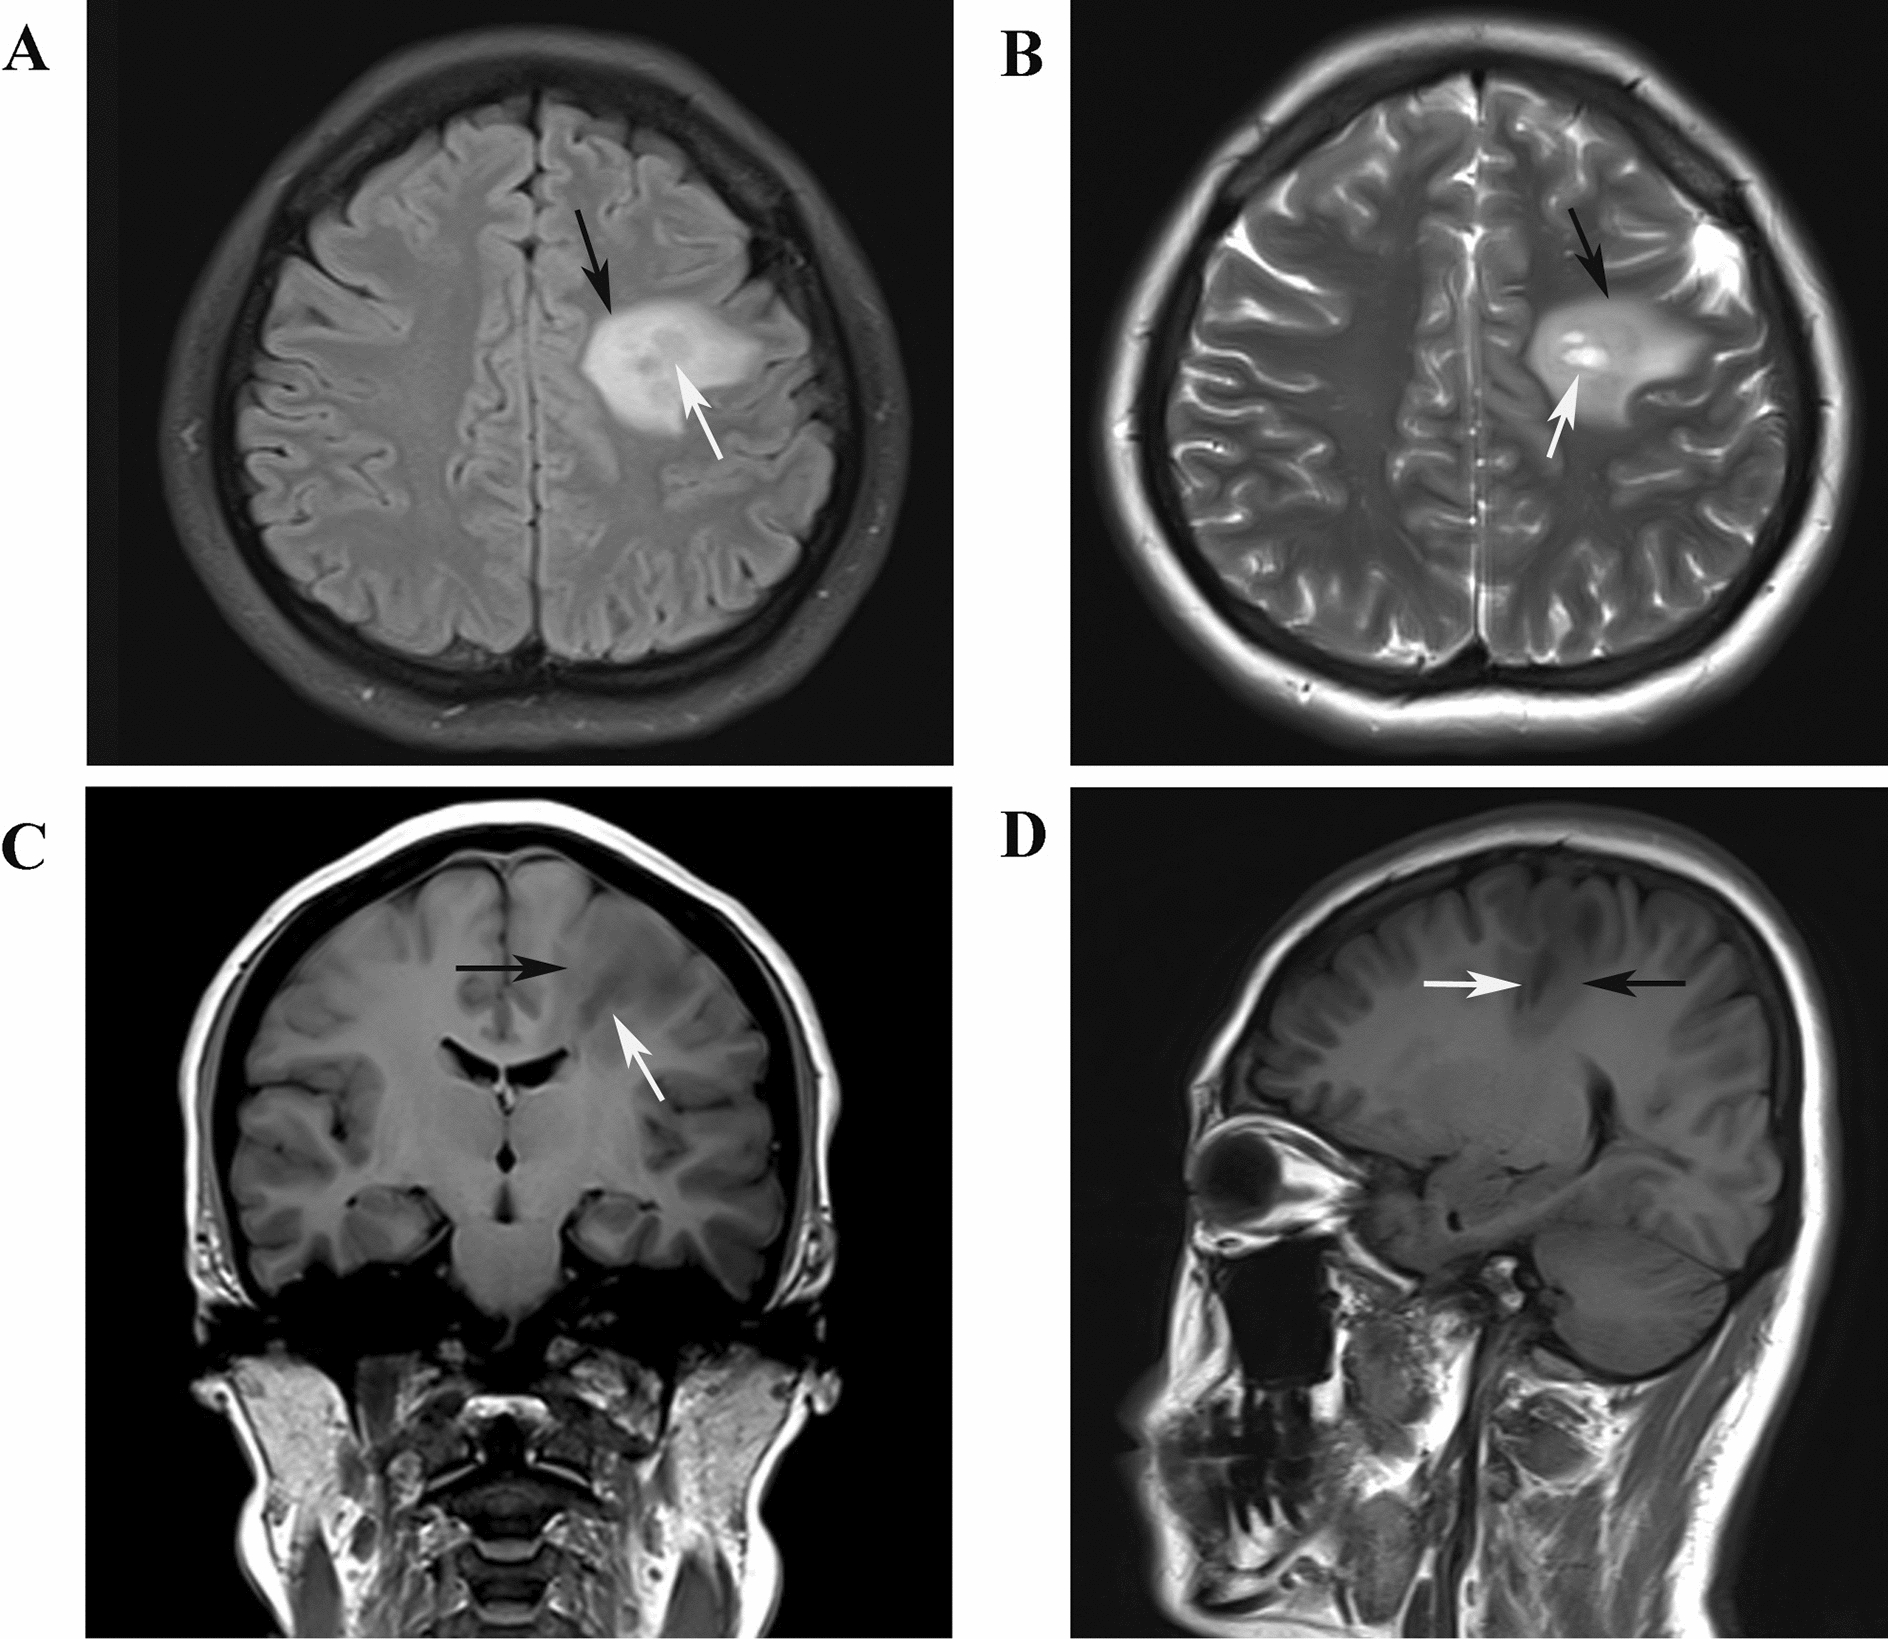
MR images before surgery show an area of inhomogeneous hypointensity on a T1-weighted image (T1WI) and hyperintensity on a T2-weighted image (T2WI) and T1-weighted fluid-attenuated inversion recovery (FLAIR) image, with a size of 15 × 17 × 22 mm^3^ (volume 3.1 cm^3^), surrounded by edema, within the left centrum semiovale (white arrows point to the center of focus; black arrows point to the region of edema). A T1-FLAIR axial view. B T2WI axial view. D T1WI coronal view. D T1WI sagittal view
